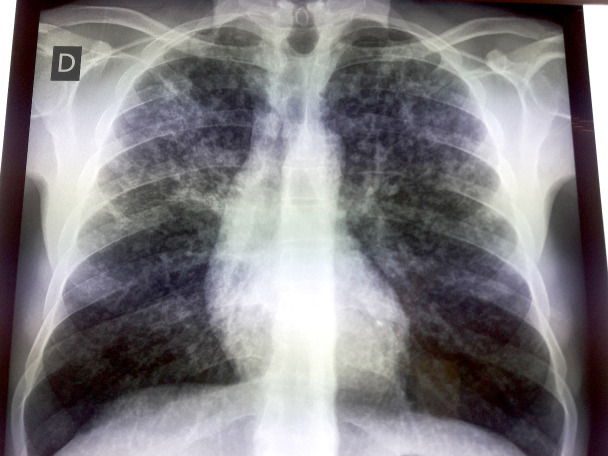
Radiographie des poumons de face, aspect de miliaire tuberculeuse Frontal chest X-ray, appearance of miliary tuberculosis
